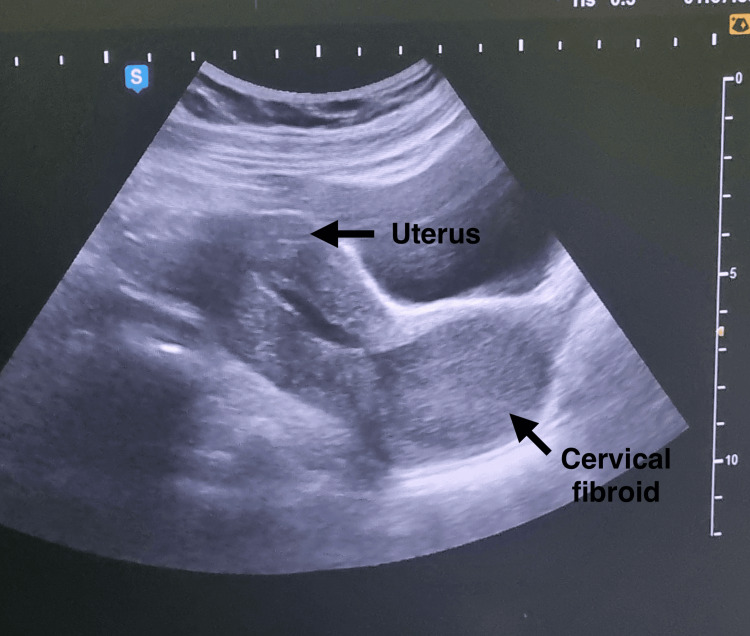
Ultrasound image showed a uterus of normal size with a well-defined heterogeneous lesion in the lower uterus and cervix, measuring 5.3 x 6.3 x 5.6 cm, provisionally diagnosed as a cervical fibroid
